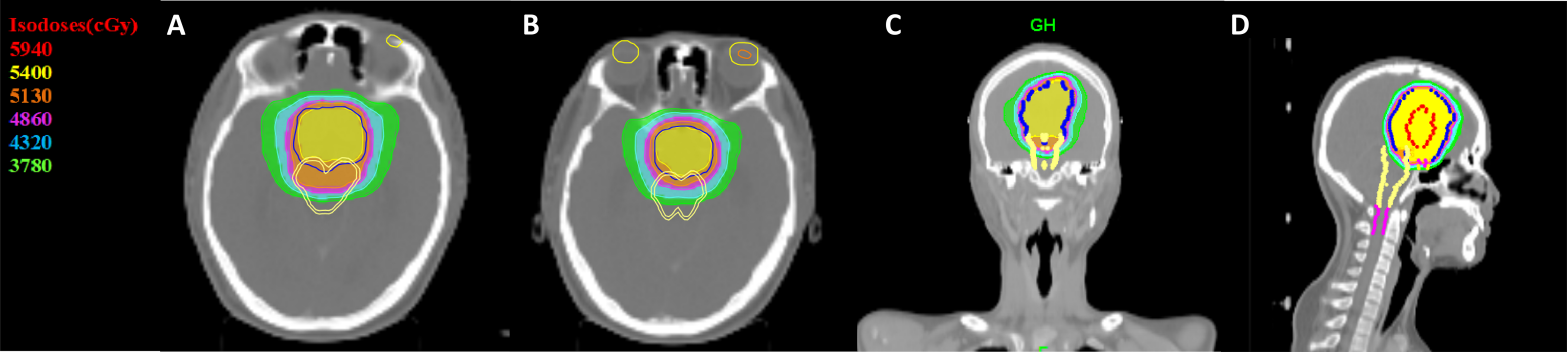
Dose distribution in a CN patient treated with IMRT. Color-wash areas: 59.40Gy (red), 54.00Gy (yellow), 51.30Gy (orange), 48.60Gy (purple), 43.20Gy (blue), 37.80Gy (green). CN, central neurocytoma; HT, Helical tomotherapy. (A, B) refer to cross section CT, (C) refer to coronal CT and (D) refer to sagittal CT.
